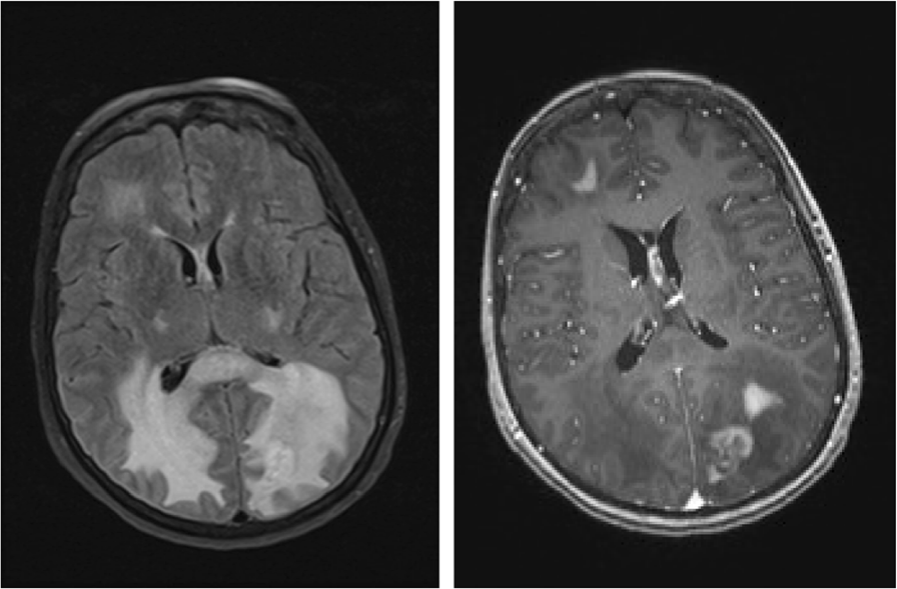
Brain MRI (October 2009): axial FLAIR (left) and axial T1 post contrast (right) revealing right frontal and left parieto-occipital enhancing lesions with surrounding edema
